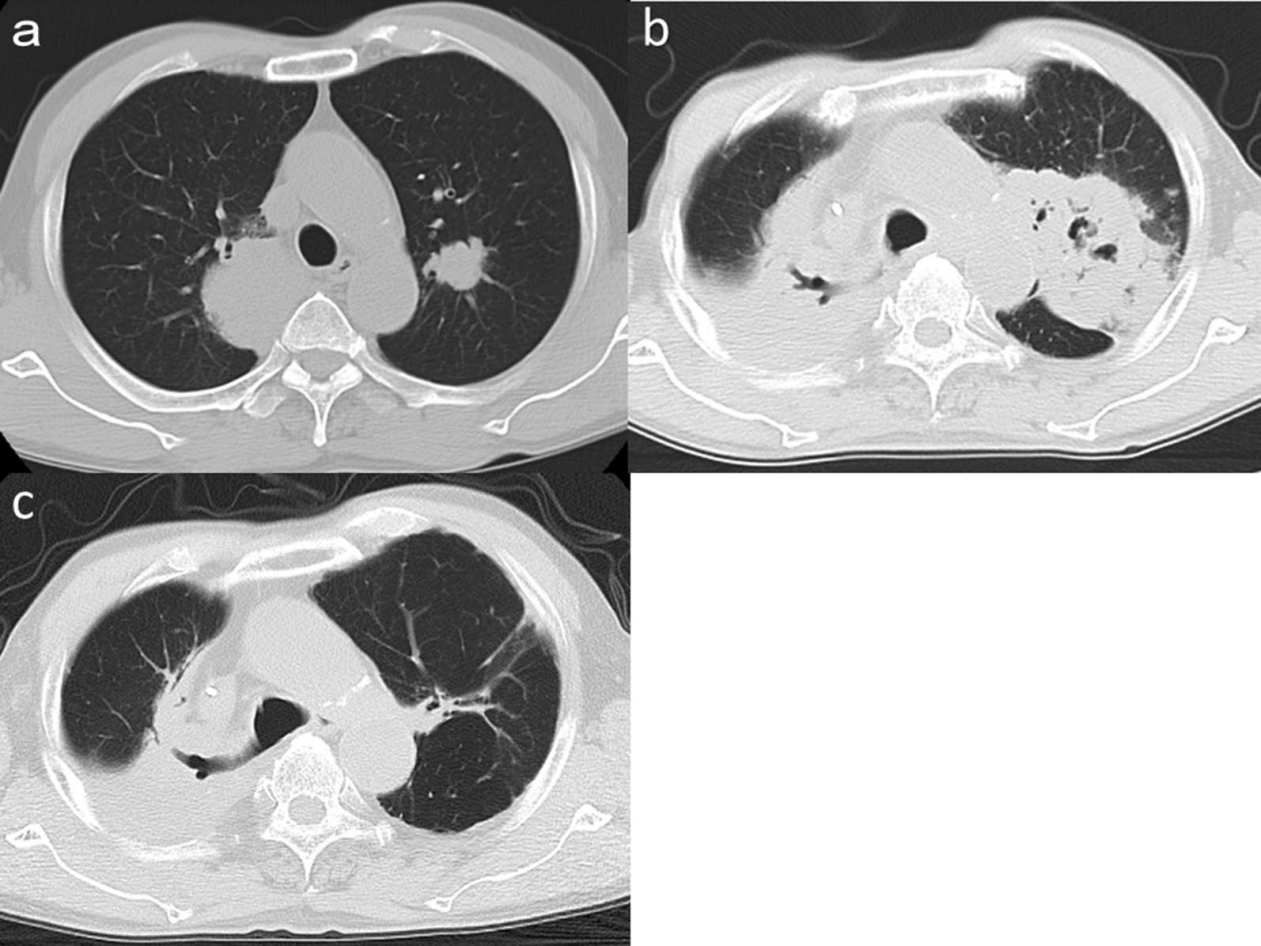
Chest computed tomography findings. a Pretreatment, masses of size 5.2 × 4.0 cm in the right upper lung lobe and 2.3 × 2.2 cm in the left upper lung lobe. b Before nivolumab, the mass in the left upper lobe grew up to 7.7 × 5.7 cm. c After nivolumab, the mass in the left upper lobe disappeared. An atelectasis remained in the right upper lobe
